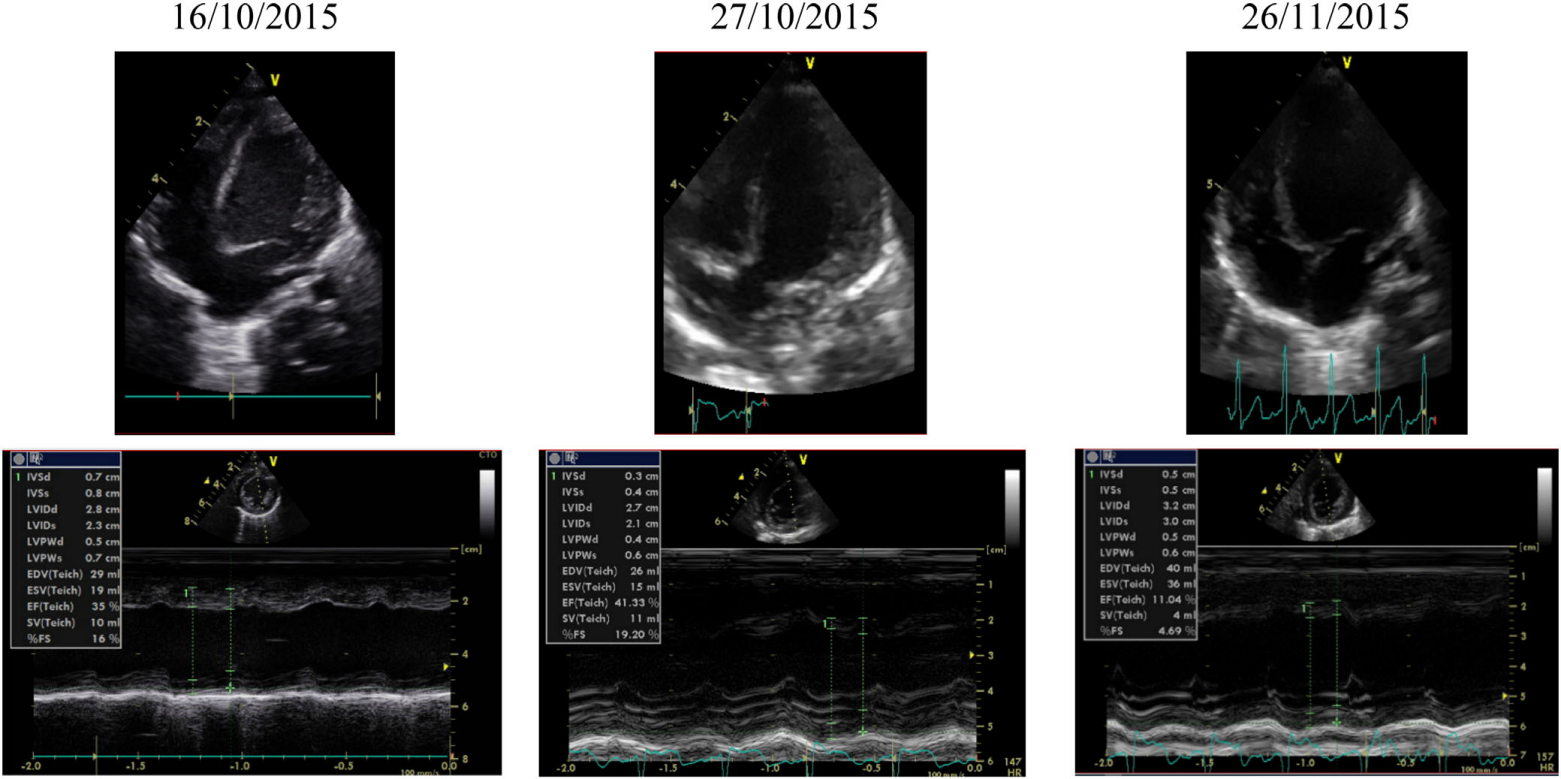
Serial echocardiographic findings during follow‐up of the described patient. Cardiac decompensation, secondary to the dilated cardiomyopathy, was rapidly progressing. Fractional shortening varied between 16% and 20% at the age of 2 months and between 4% and 11% at the age of 3 months.
